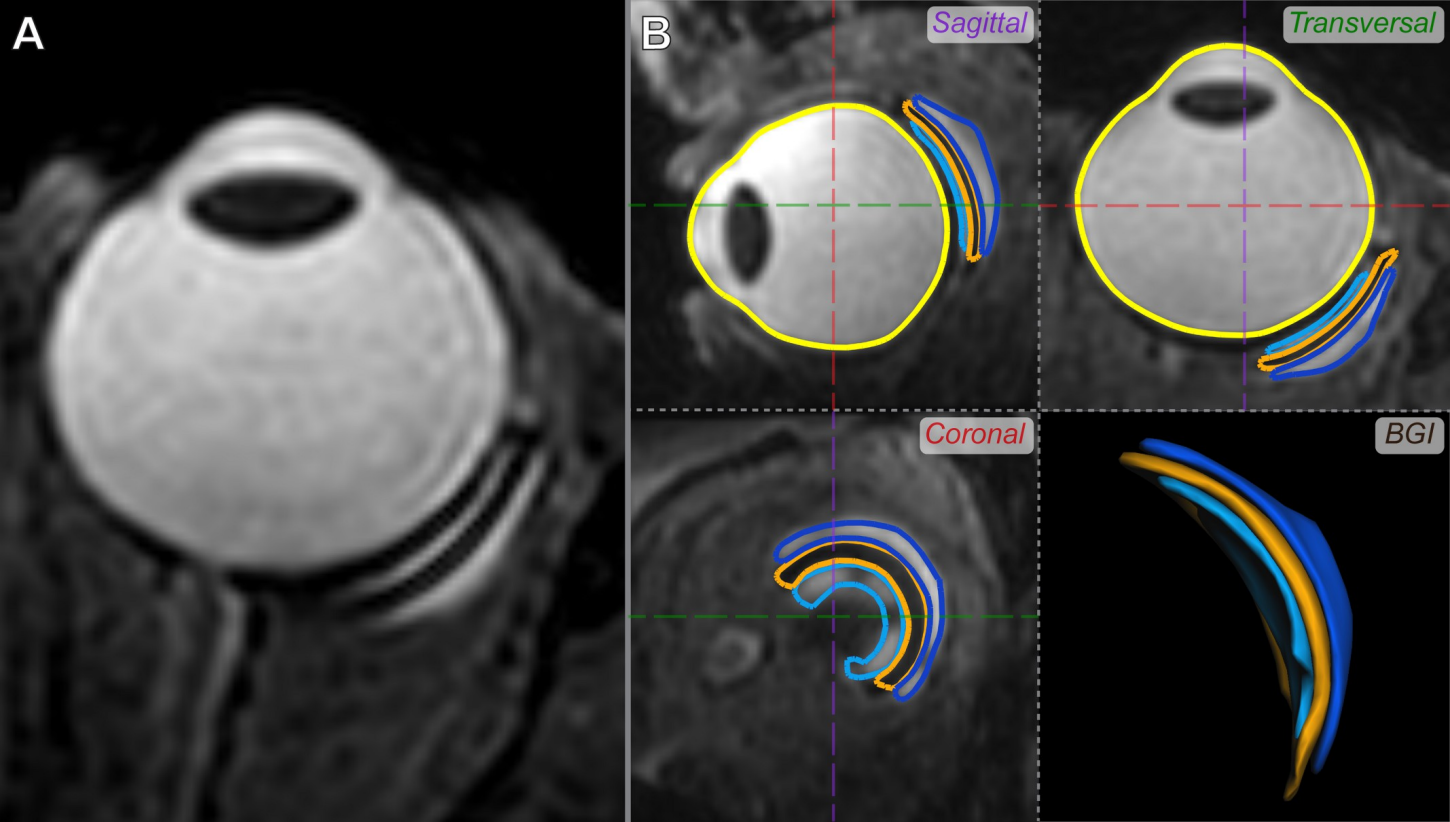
Three-dimensional MRI assessment of the BIG. A) Typical 3D T2-weighted MR-image of an eye with a BGI. B) Volumes segmented from that same MR-Image in three orthogonal reformats (sagittal, transversal and coronal), allowing for an accurate and 3D assessment of the bleb and its relation to the eye. Displayed are the inner bleb *(dark blue)*, BGI *(orange)* and outer bleb *(light blue)*. A segmentation of the eye *(yellow)* is also shown for reference.
